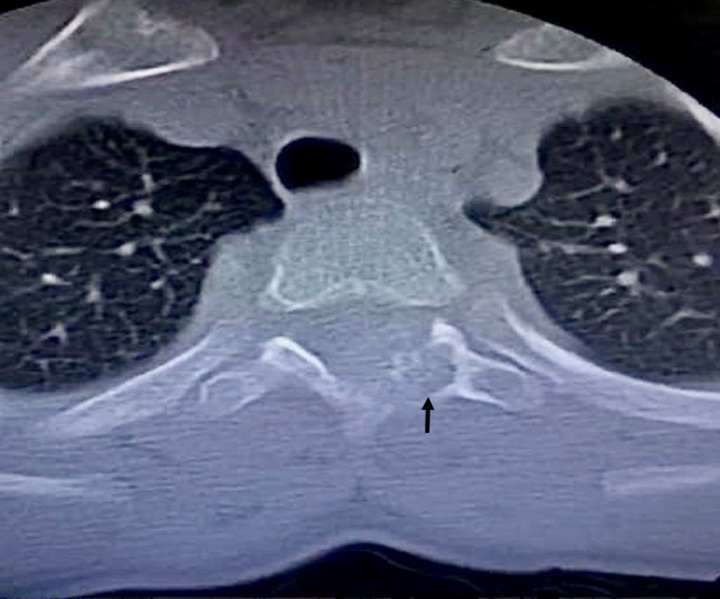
The axial computed tomography scan image of the thorax demonstrated an aggressive lytic lesion (arrow), expanding and scalloping of the posterior pedicles of thoracic vertebrae (T3–T4 level).
